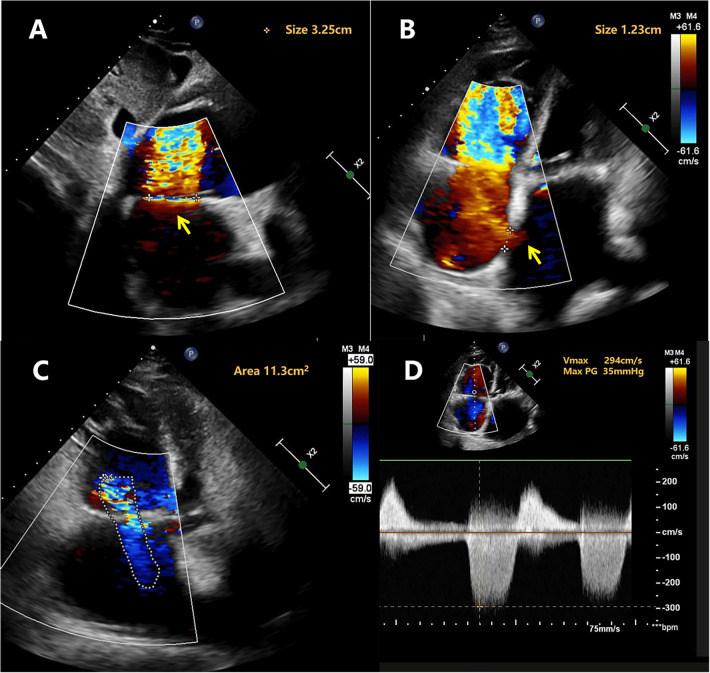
Echocardiographic findings demonstrating the atrial septal defect with significant left-to-right shunting. (A) Extensive left-to-right flow across the mid-atrial septum, with a defect length of approximately 3.25 cm; (B) Maximal shunt orifice measuring approximately 1.23 cm; (C) Tracing of the atrial septal defect region showing an effective shunt area of approximately 11.3 cm^2^; (D) Pulsed-wave Doppler across the tricuspid level showing a peak shunt velocity of approximately 294 cm/s, corresponding to a pressure gradient of about 35 mmHg.
